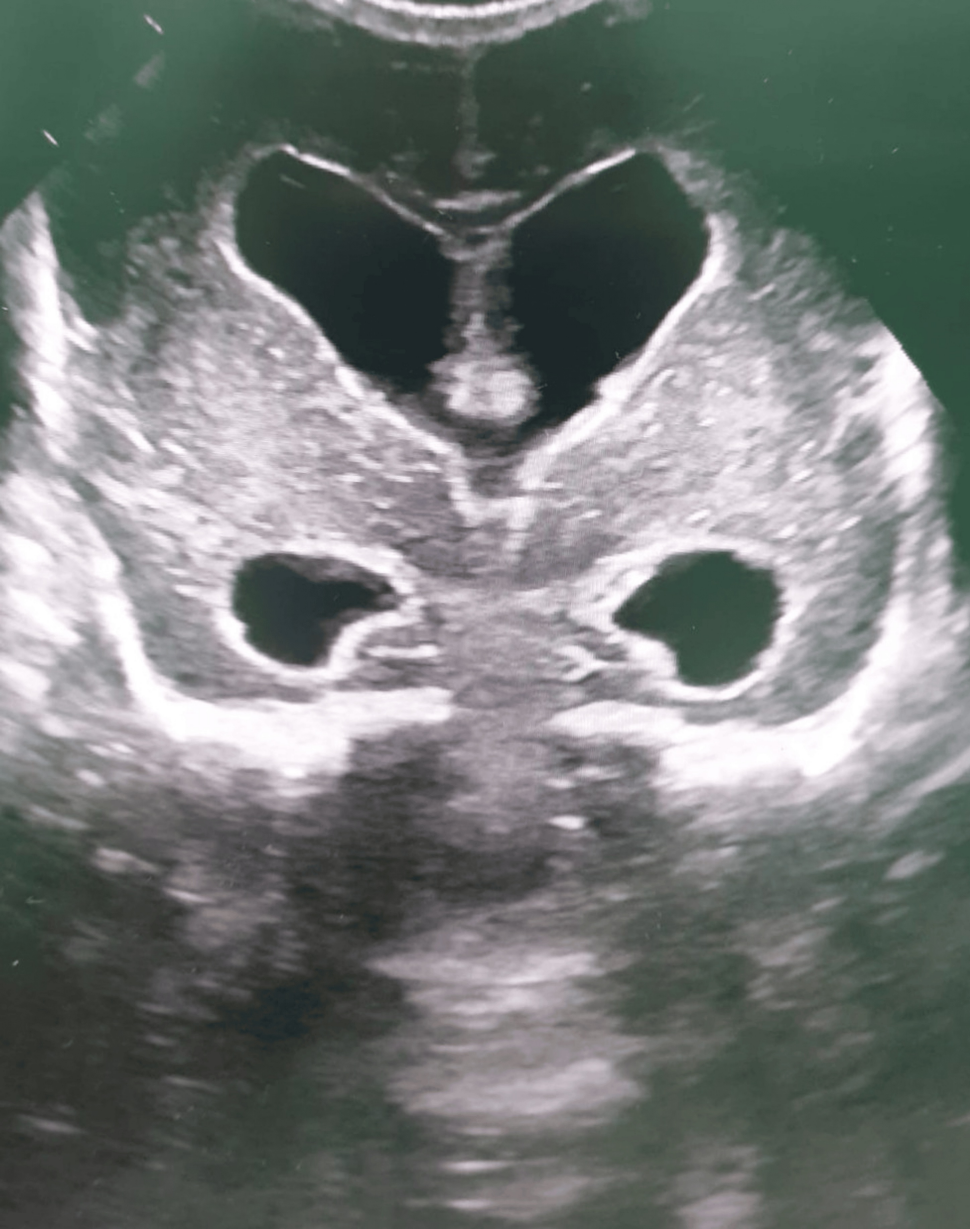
Cranial ultrasound of twin 2 demonstrating severe intraventricular hemorrhage with ventriculomegaly.
